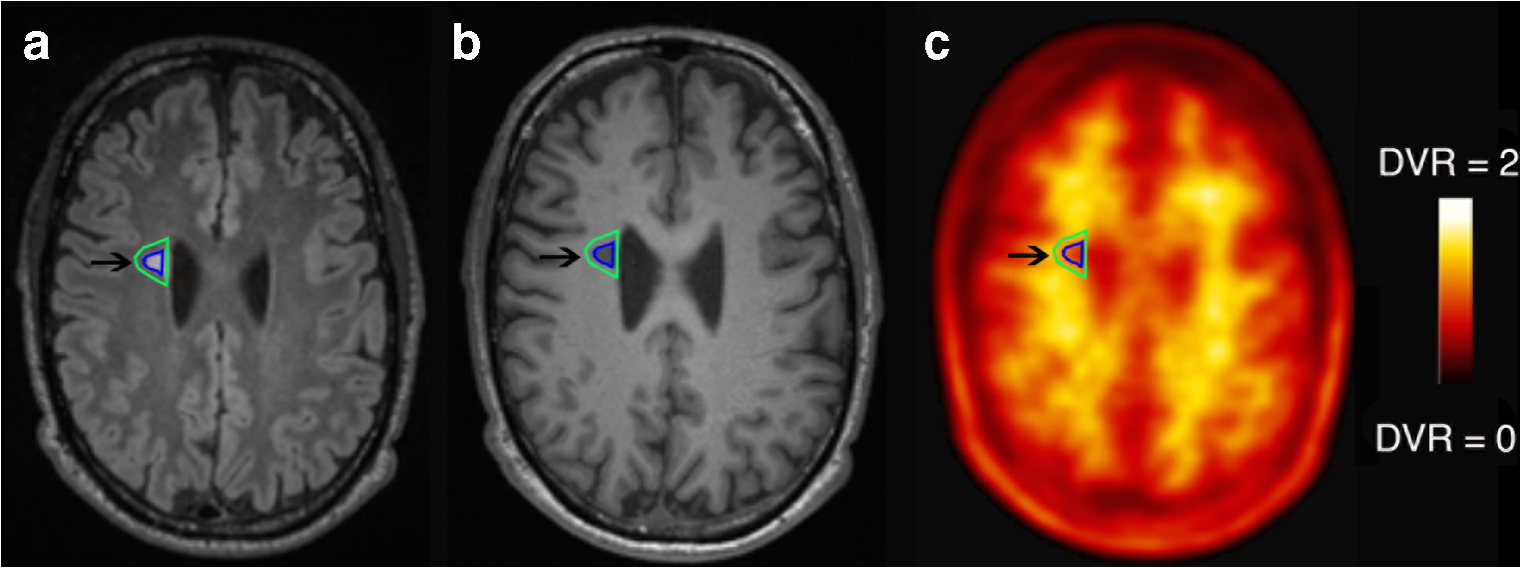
[^18^F]Florbetapir PET and MRI images from a Multiple Sclerosis patient. 3D-T1w MPRAGE image (a), FLAIR image (b) [^18^F]florbetapir distribution volume ratio (DVR) parametric map (c) for a 54-year-old female relapsing-remitting Multiple Sclerosis patient (disease duration = 10 years; Annualized-Relapse-Rate of 0.3; EDSS = 3.5; and MSSS = 4.55). Arrows point to T1 lesions (a) and T2 lesions (b) showing a reduced [^18^F]florbetapir binding in the DVR parametric map (c). Colour bar reflects range of [^18^F]florbetapir DVR. Arrows point to demyelinating lesion, with both the lesion (in blue) and perilesional rim (in green). In perilesional area (up to 8 mm) DVR values were reduced compared with the white matter as defined through standard MRI in healthy controls (estimated 7% loss, *P* < 0.01)
